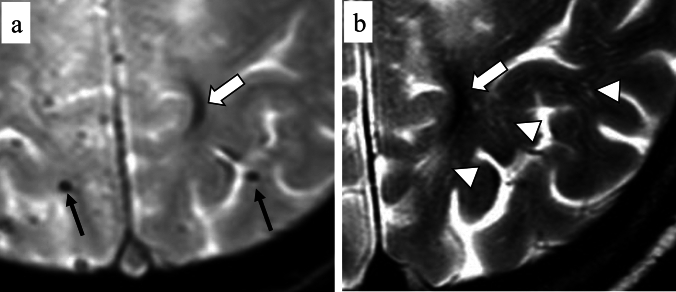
A 69-year-old female with probable CAA. MMSE score is 21. T2*-weighted image (a) and T2-weighted image (b) show hypointensity in the left parietal gyrus, indicating an intragyral hemorrhage (arrow). In addition, enlarged PVS (arrowheads) are observed around the intragyral hemorrhage on T2-weighted image (b). CMBs in bilateral parietal lobes are also depicted (small arrow) on T2*-weighted image
